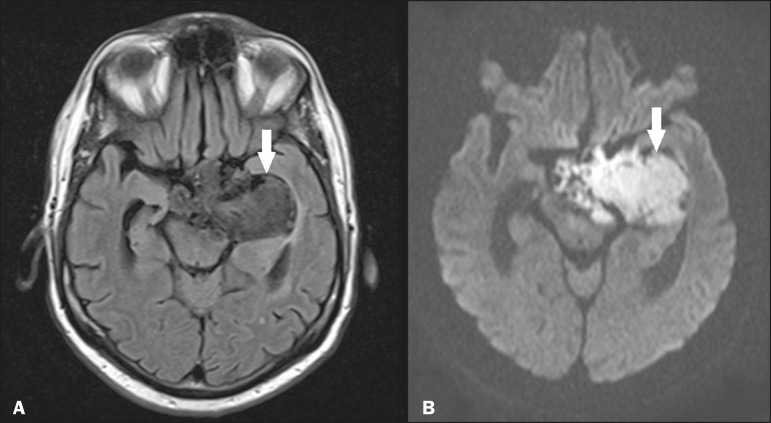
Epidermoid cyst. MRI showing a cyst-like formation in the suprasellar and left juxtasellar region, showing a hypointense signal in a FLAIR sequence (A - arrow) and a hyperintense signal in a functional diffusion-weighted sequence (B - arrow).
